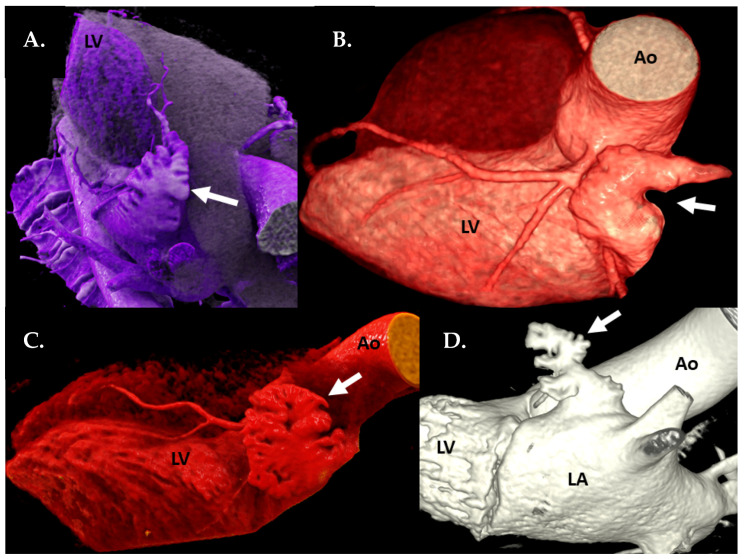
Computed tomography angiography (CTA): 3D volume rendering technique (VRT) reconstructions of the 4 LAA types (white arrows): (A) cactus, (B) chicken-wing, (C) cauliflower, (D) windsock. Ao—ascending aorta, LA—left atrium, LV—left ventricle.
